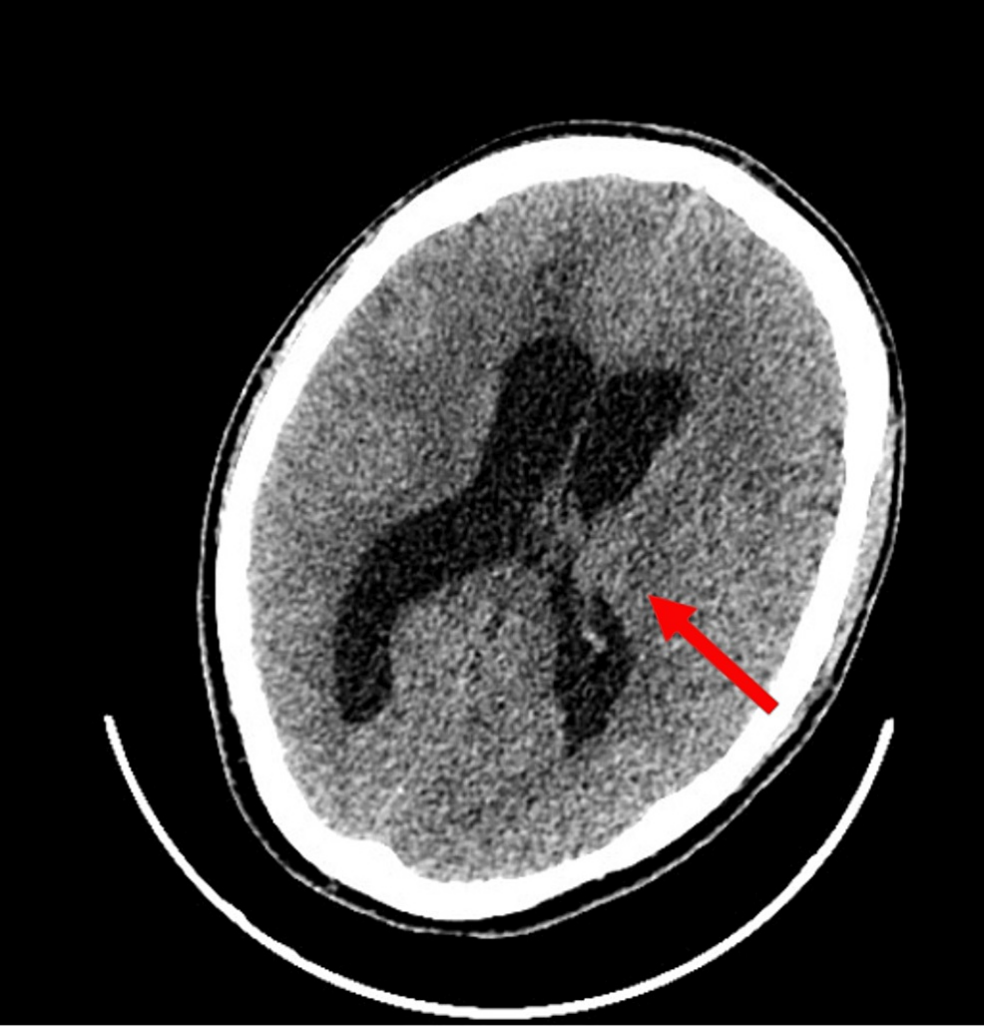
CT scan showing hydrocephalus on day 42 of the hospitalization
